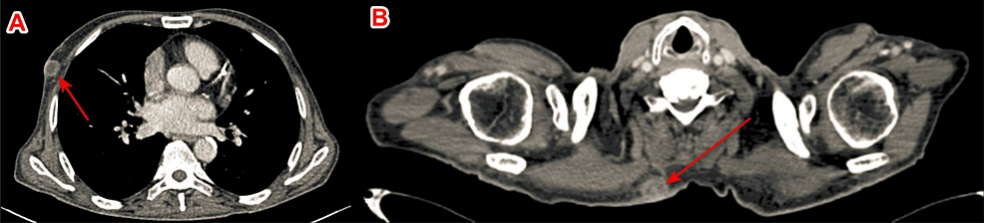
Axial section of cervical and thoracic CT scan showing cutaneous metastases in the right breast region (A) and posterior cervical region (B) CT: computed tomography
